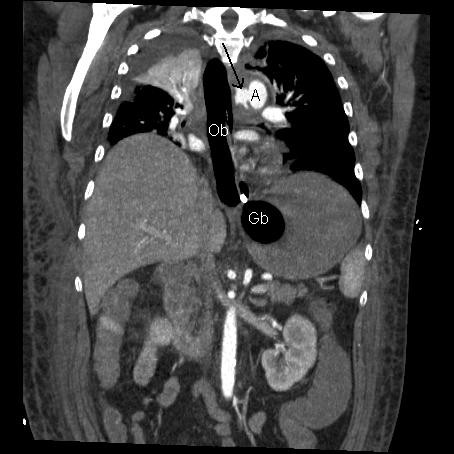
CT angiogram – coronal view. The oesophageal balloon (Ob) and gastric balloon (Gb) from the Sengstaken-Blakemore tube are easily visible. The black arrow points to the leakage of contrast from the aorta (A) indicating a breach in the aortic wall.
